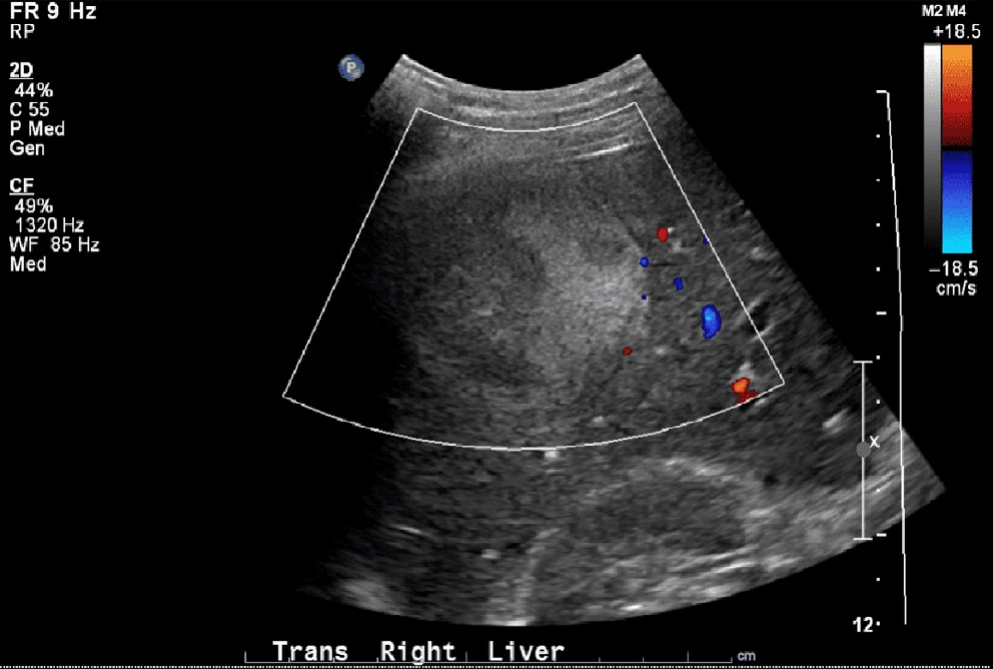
Ultrasound Doppler of the larger liver mass in segment VI measuring 5.9 × 5.3 × 5.5 cm in size and with peripheral blood flow
